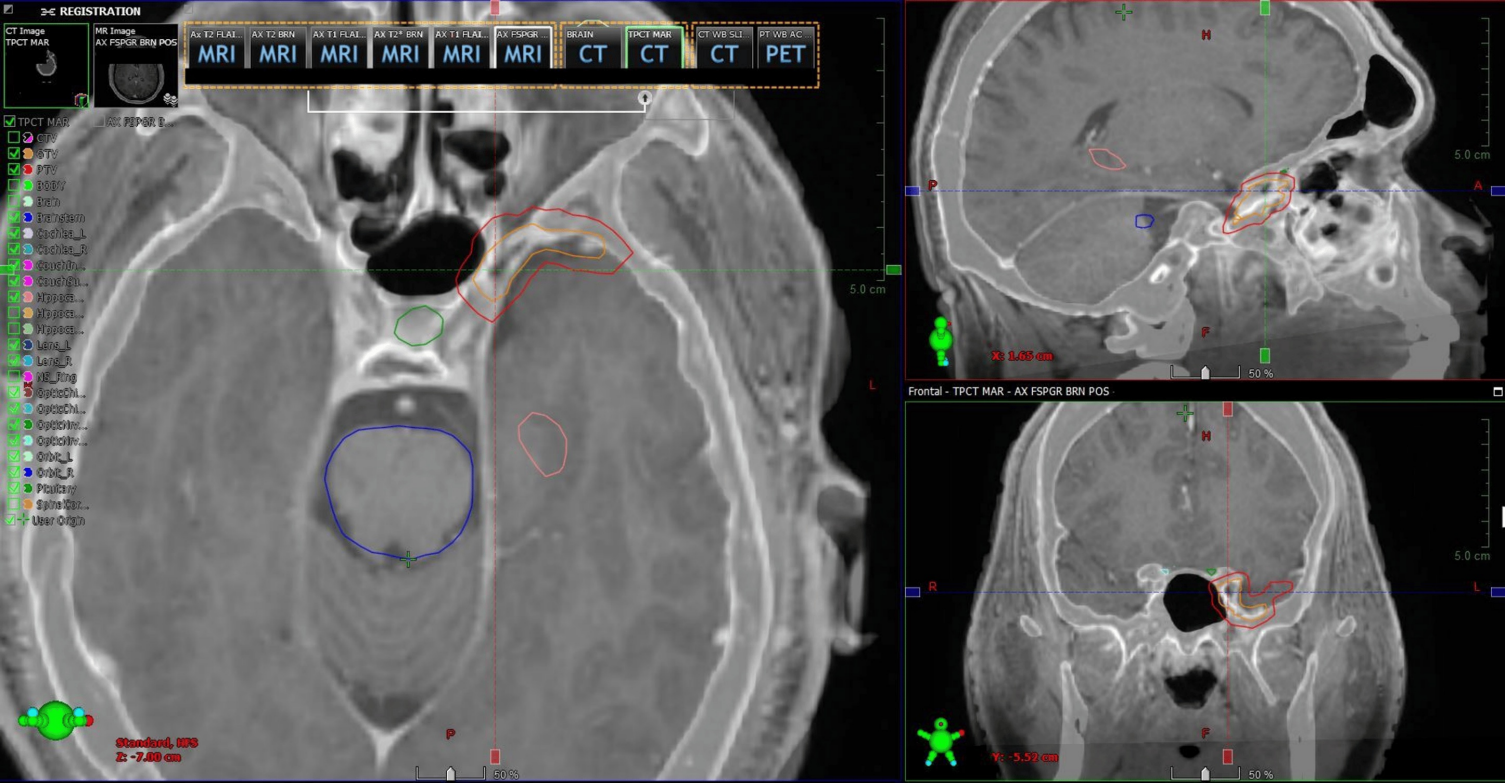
CT/MRI fusion before obtaining 68Ga-DOTATATE PET. Co-registered T1 post-contrast MRI and contrast-enhanced CT images showing accurate alignment of the left cavernous sinus lesion and adjacent skull base structures for subsequent molecular imaging registration.
